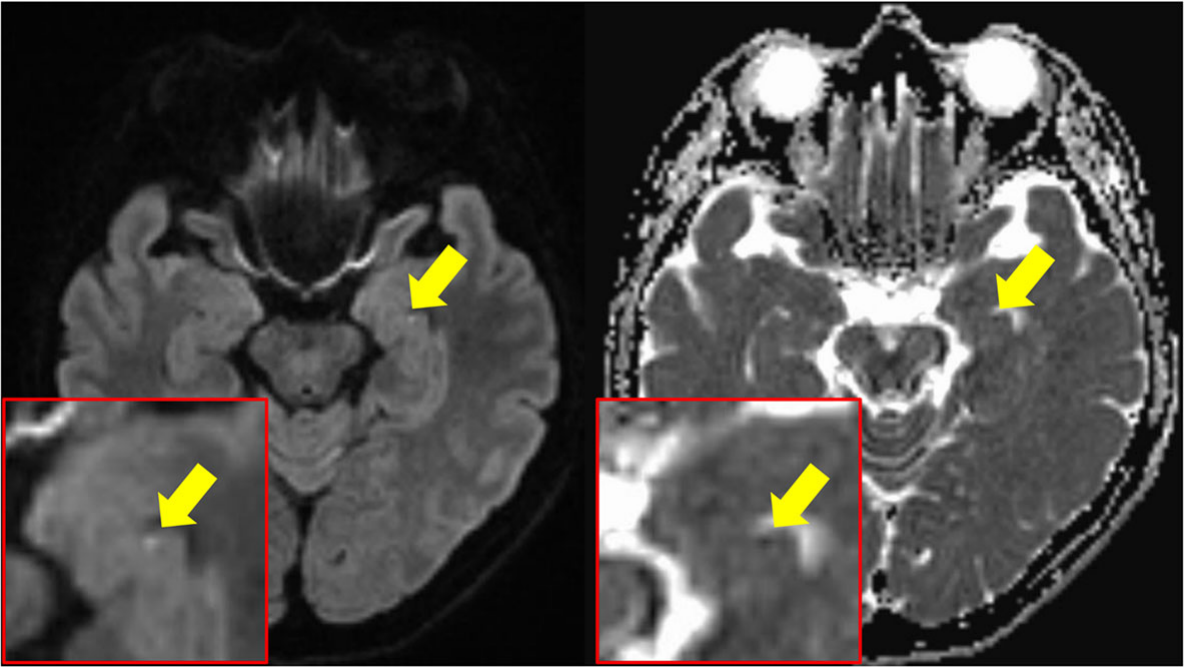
Brain magnetic resonance imaging (MRI) showed a high-density spot in the left hippocampus on diffusion-weighted imaging at the first admission
